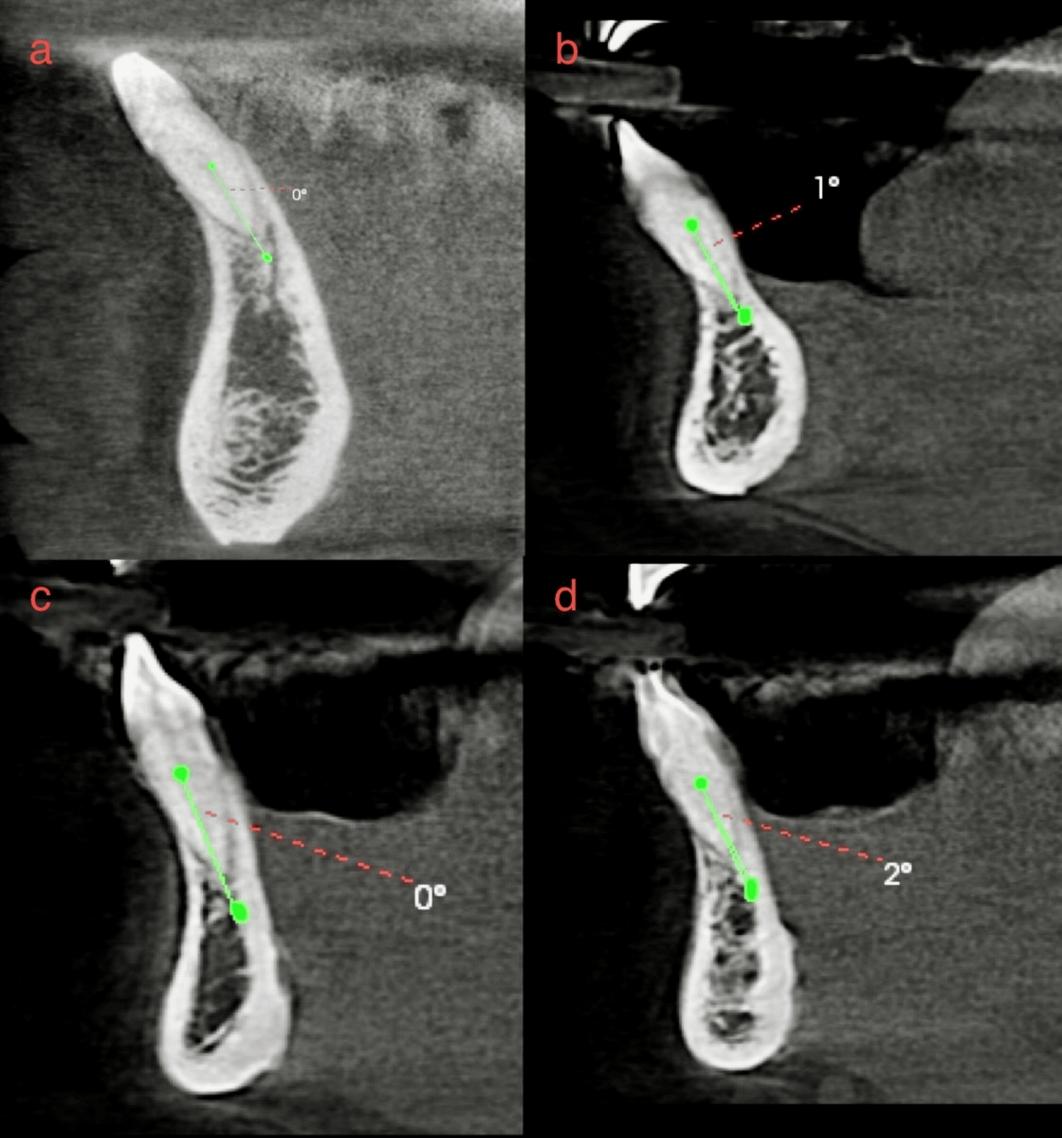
(a-d) Four different CBCTs in which the external root curvature was determined using Schneider's Classification and found to be straight as the angle was <5 degrees. CBCT - Cone beam computed tomography
